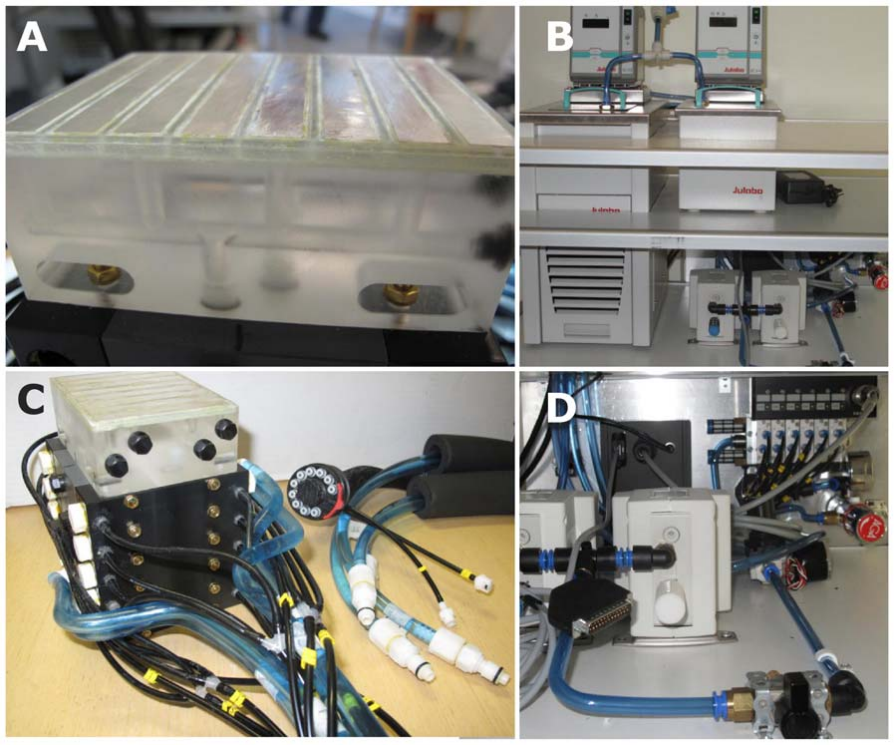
The thermal grill-unit. Panel A) Detail of the surface of the thermode, consisting of eight silver bars each measuring 11 mm×80 mm. The bars were mounted onto a Perspex-block bringing odd and even numbered bars into direct contact with (potentially) different pools of circulating water. Channels drilled through the Perspex-block connected to the inlet/outlet unit (grey-colored block) through the bottom of the thermode. Panel B) Water baths for cooling and warming, connected to a circulation pumps. Panel C) The TGI-thermode attached to the grey Delrin inlet/outlet unit. The Delrin-block has bored channels in which ten pneumatically operated piston-valves operate. The two segregated pools of warm- and cold-water are continuously circulated through the unit (blue hoses). The position of the valves, set through computer controlled pneumatics (thin black tubes), determine the water-flow to the Perspex-block thermode. The thermode and inlet/outlet unit are connected to the baths, pumps and control devices through a thermally insulated umbilical-cord running through the radiofrequency wave-traps of the MRI-Faraday cage. Panel D) Pressurized air-operated process pumps for circulating the water, along with computer-controlled pneumatic switches – allowing precise timing of stimulus-presentation.
